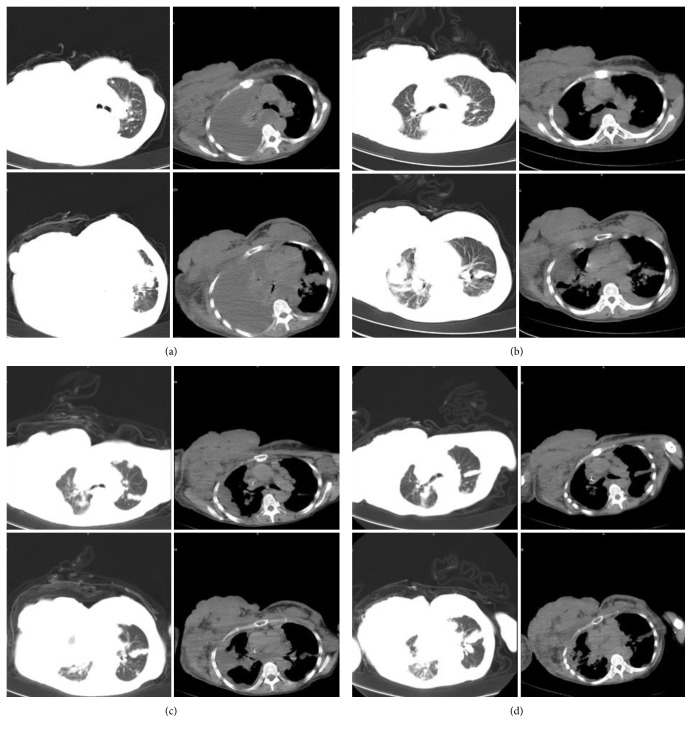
CT scan showed bilateral lung metastasis and pleural fluid. (a) Massive right pleural fluid and bilateral breast metastasis were detected before treatment on September 22, 2016; (b) massive right pleural fluid mostly disappeared, and bilateral breast metastasis was stable after three weeks of treatment, on October 14, 2016; (c) No further observation of right pleural fluid was made, and bilateral breast metastasis was stable after four months of treatment, on January 26, 2017; (d) No further occurrence of right pleural fluid or lung metastasis was noted, and bilateral breast metastasis was stable after seven months of treatment, on April 27, 2017.
